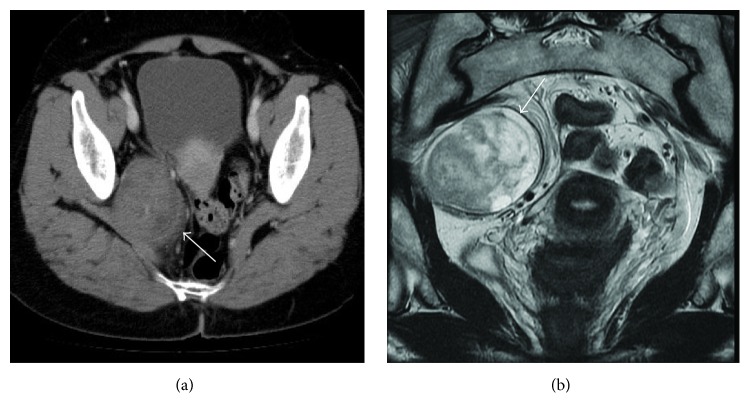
(a) Enhanced abdominal computed tomography revealing a 65 × 50 mm, solid, well-defined, heterogeneous mass (arrow) in the right lateral pelvis space. (b) Magnetic resonance imaging revealing heterogeneous hyperintensity in the tumor (arrow) on T2-weighted image.
